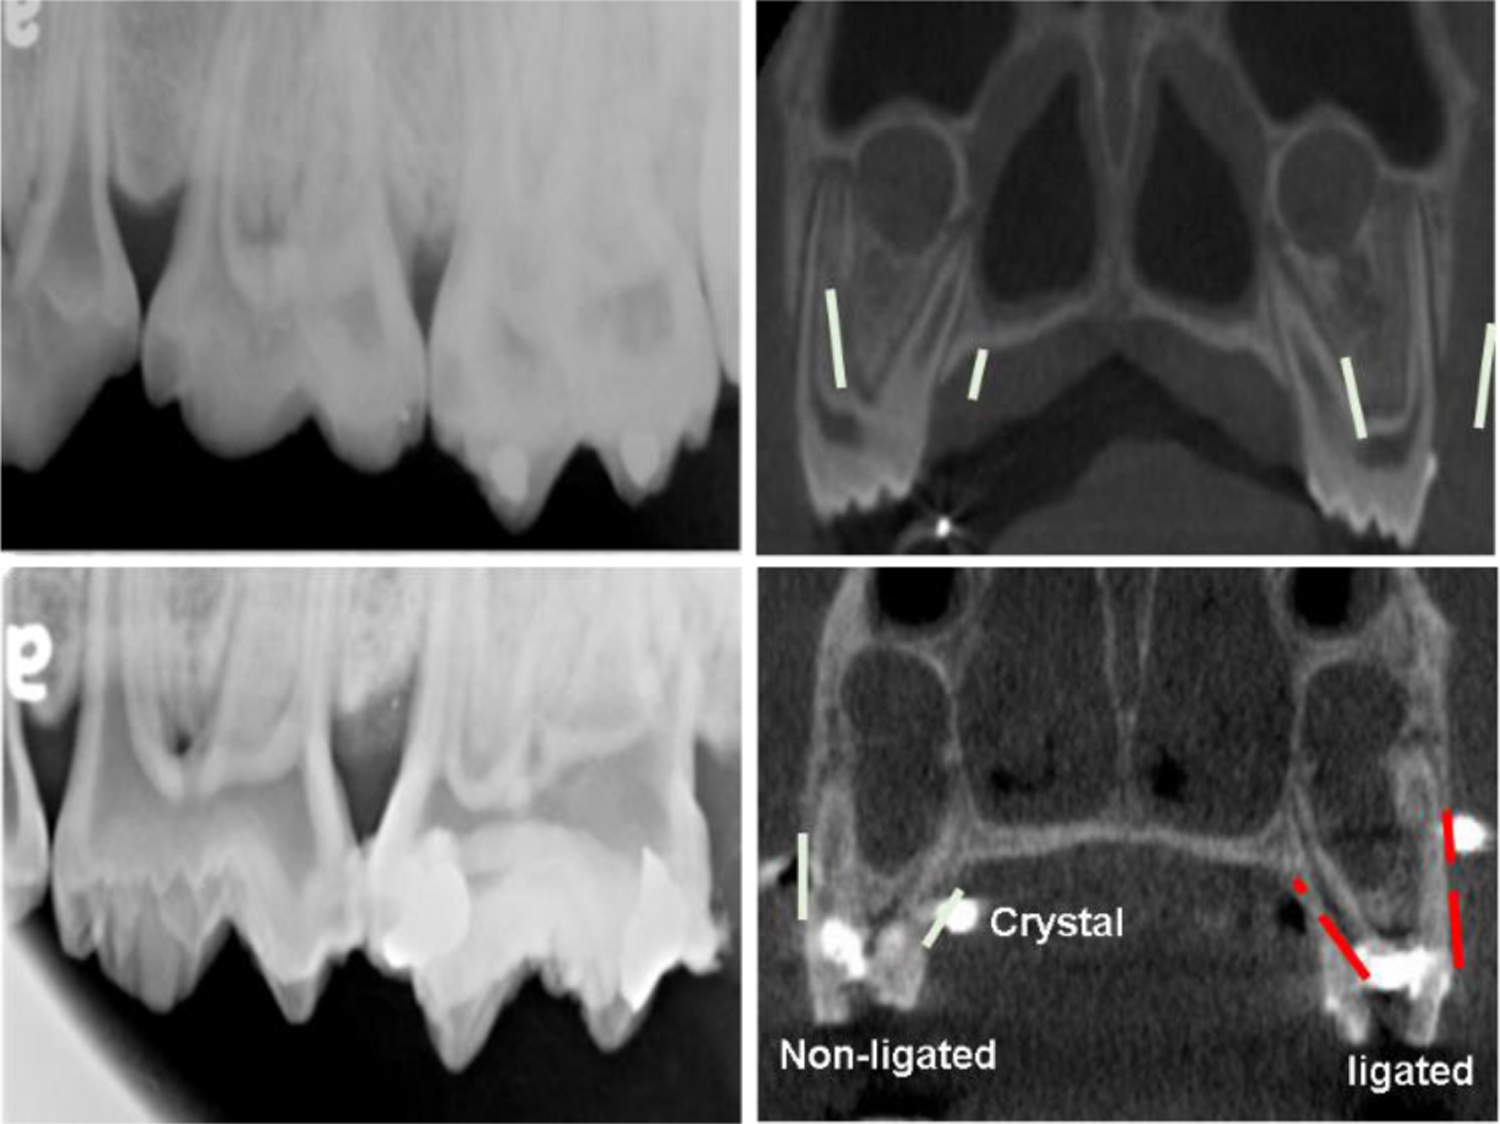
Comparisons of alveolar ridge height between baseline (upper row) and 8 weeks after experimental periodontitis induction (lower row) in both PA (left column) and CBCT images (right column).
